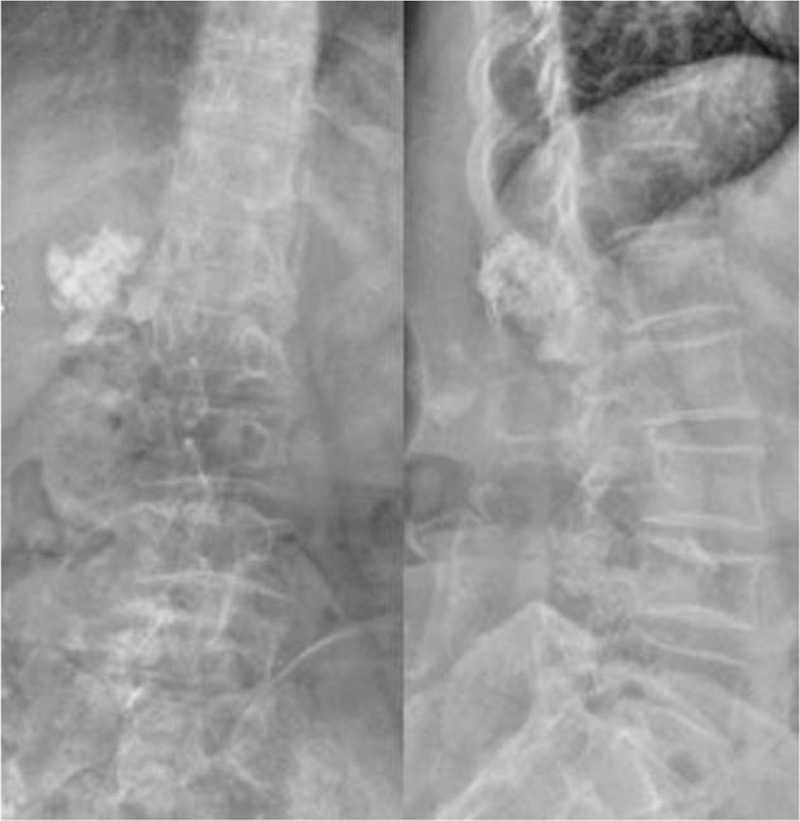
X-ray examination showed an irregular, high-density shadow in the posterior aspect of L1-2.
